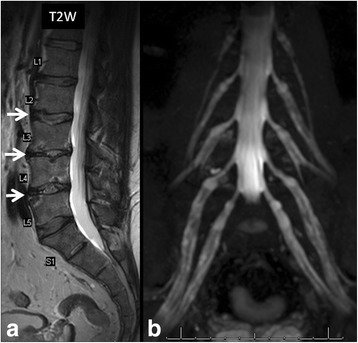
64-year-old man with diabetes mellitus with low back and bilateral leg pain consistent with diabetic neuropathy. Sagittal T2-weighted image (a) shows end plate fragmentation at multiple lumbar levels (L2/3, L3/4, L4/5, *arrows*). MR neurography image (b) shows diffuse lumbosacral plexopathy with patchy thickening of all nerves. The patient showed no clinical evidence of active infection
